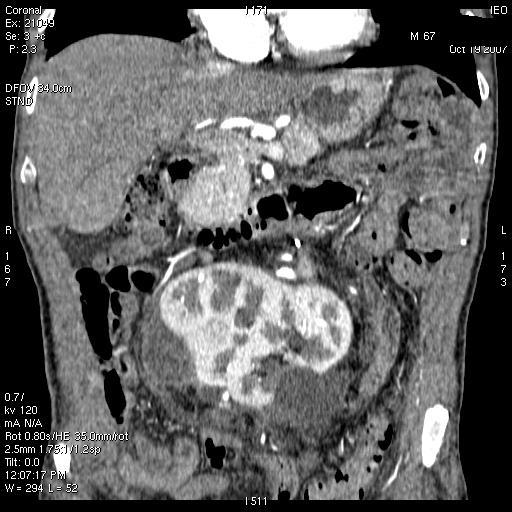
Abdominal CT after surgery—patient 1.
